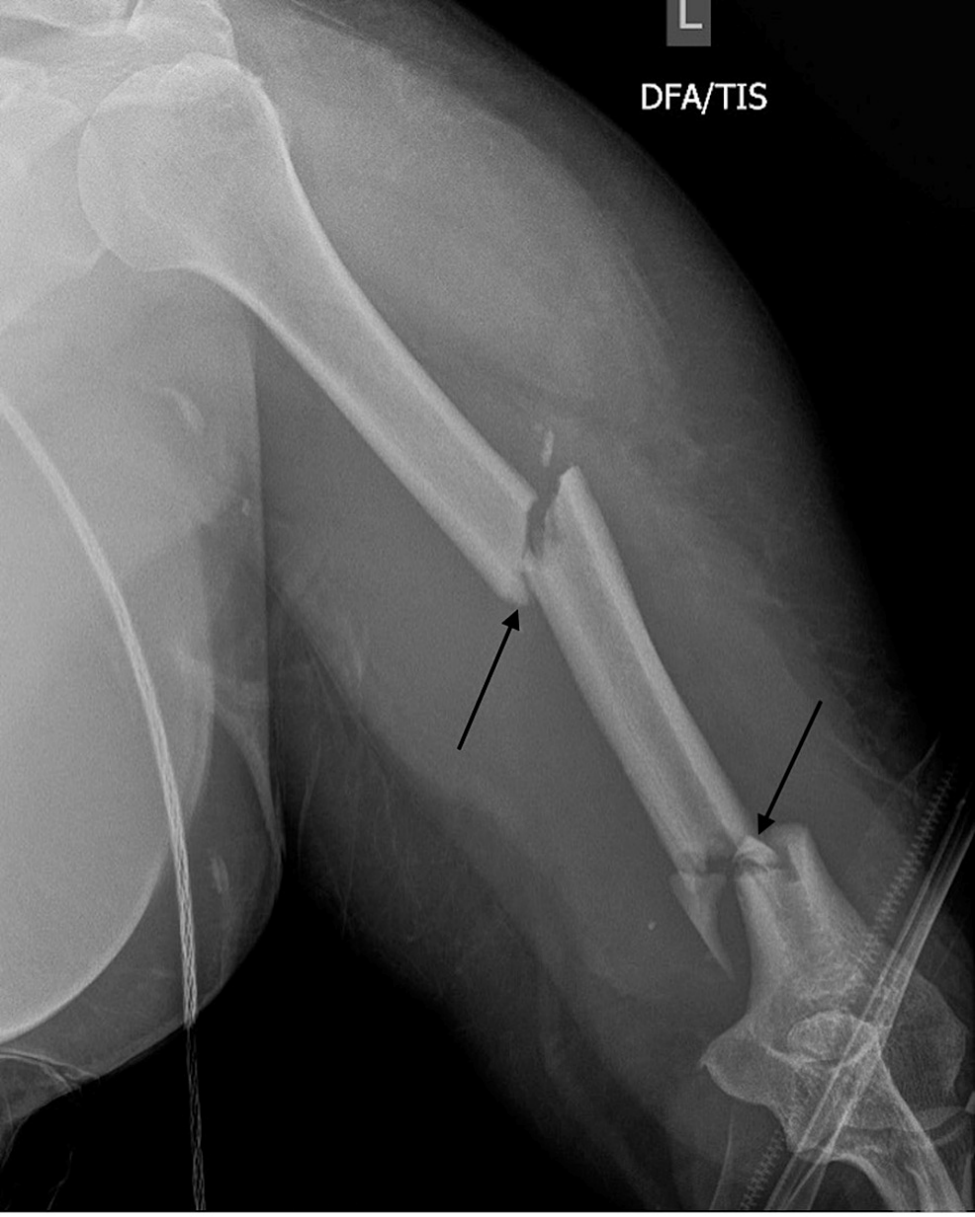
Anterior/posterior radiographs of a humerus demonstrate an example of a segmental fracture pattern with arrows to show the separate fractures.
